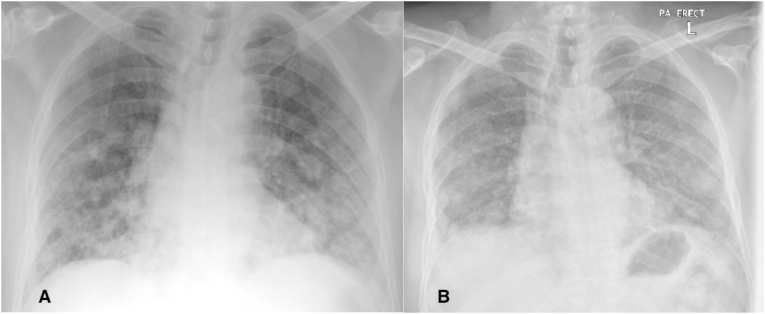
(A and B): Chest X-Ray showing bilateral pulmonary nodules. (A) The new film upon presentation. (B) 2-years before.
